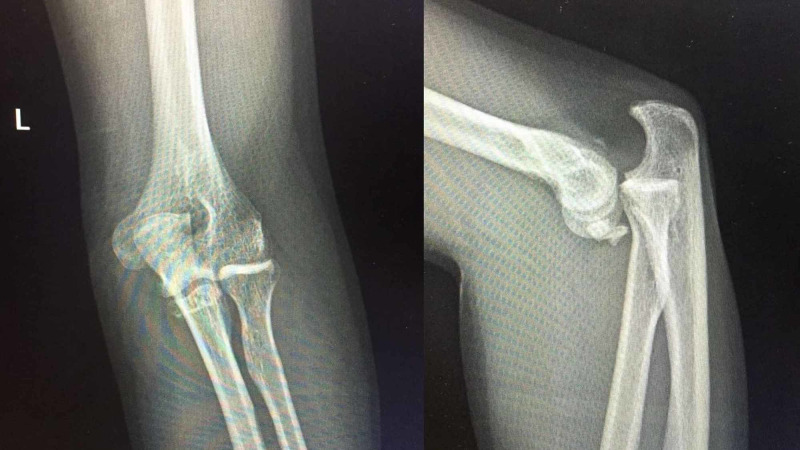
Case 5 - anteroposterior and lateral radiographs of the elbow showing a coronoid fracture with elbow dislocation
